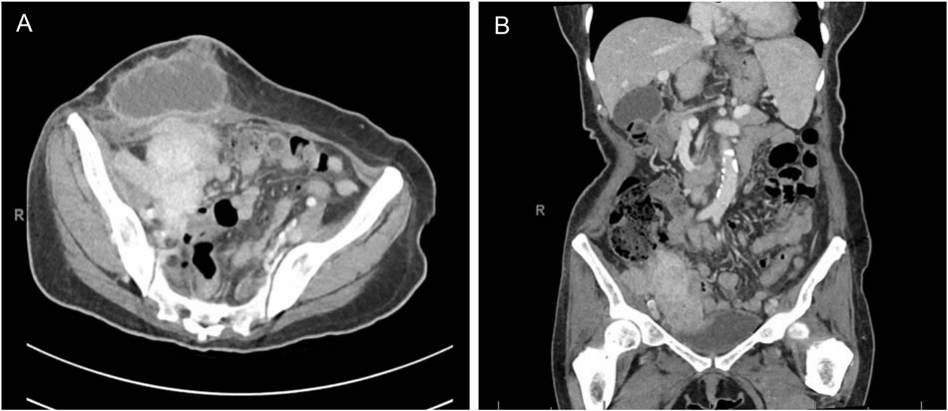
Computed tomography of the abdomen and pelvis in the axial (A) and coronal (B) planes demonstrating a 7.8 × 4.3 cm mass along the right pelvic sidewall and necrotic mass in the anterior abdominal wall with a cutaneous fistula.
